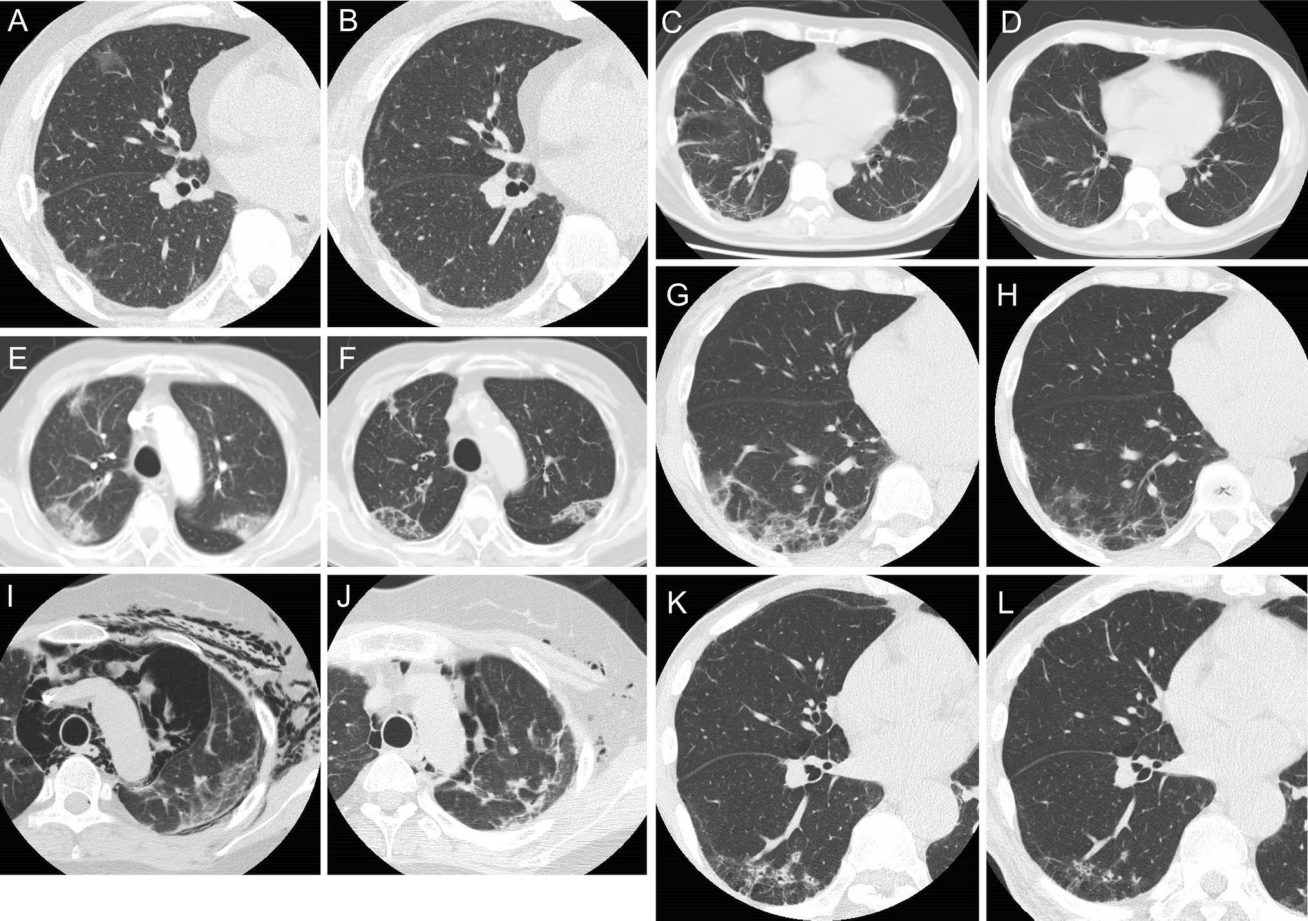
Chest computed tomography imaging. Case 1: A before and B 2 weeks after baricitinib initiation; Case 2: C before and D 5 days after baricitinib initiation; Case 3: E before and F 4 weeks after baricitinib initiation; Case 4: G before and H 4 weeks after tofacitinib initiation; Case 5: I before and J 5 weeks after tofacitinib initiation; Case 9: K before and L 5 weeks after tofacitinib initiation
